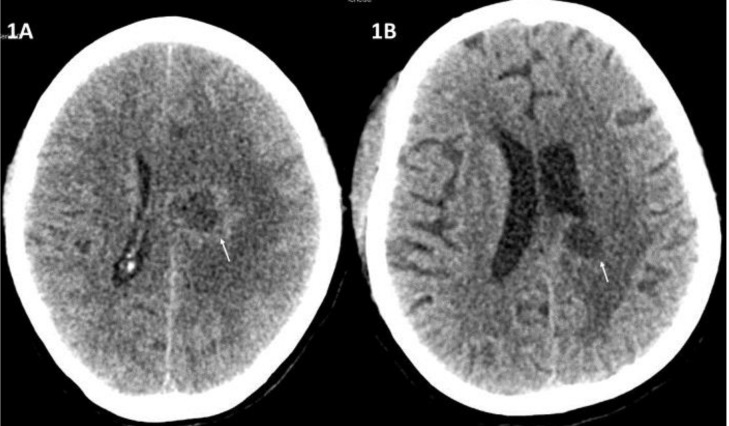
Contrast-enhanced computed tomography (CECT) axial sections of the brain showing a well-defined ring-enhancing lesion (arrow) in left centrum semiovale with perilesional oedema (1A). CECT brain post-treatment showing a significant decrease in the size of the lesion (arrow) as well as perilesional oedema (1B).
